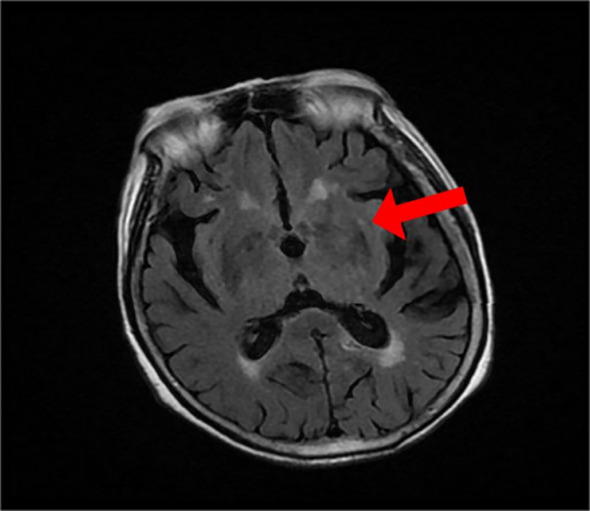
T2-weighted imaging (T2WI): Corresponding area shows slightly hypointense signal (indicated by red arrow), visible high signal in the brain white matter.
